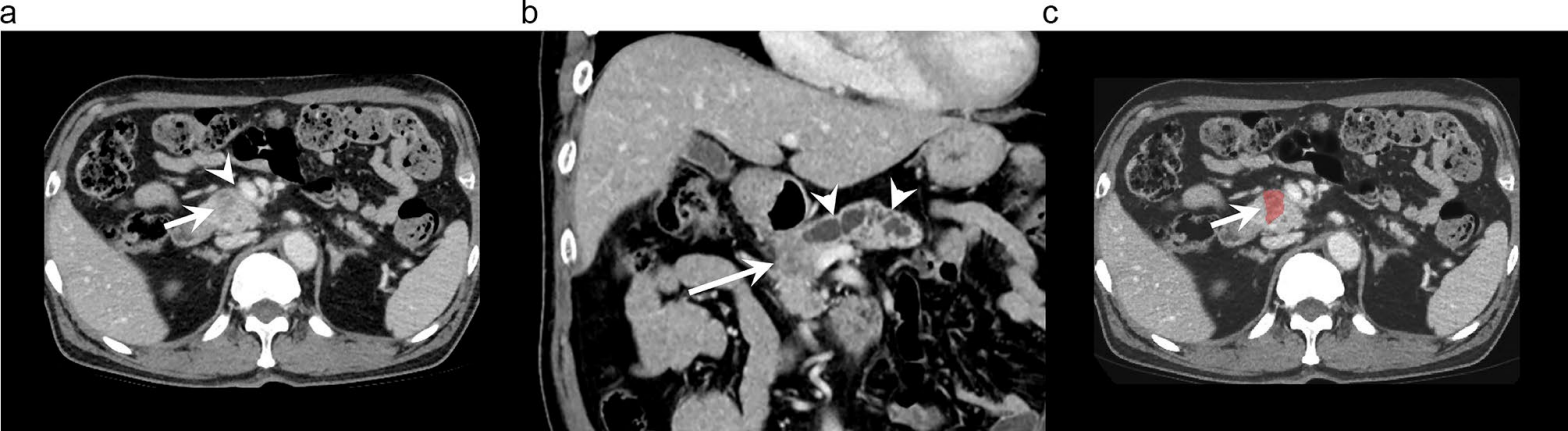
CT images of a 64-year-old male with pancreatic cancer. The patient’s preoperative CA19-9 level was 16.7 U/ml. (a) Axial portal venous phase CT image shows a 3.2 cm-sized pancreatic cancer (arrow) at the pancreas head. There was abutment of the tumor to the superior mesenteric vein (arrowhead), but no evidence of adjacent organ invasion. (b) Coronal portal venous phase shows the pancreatic cancer (arrow) with upstream main pancreatic duct dilatation (arrowheads). (c) Portal venous phase with segmentation overlay. An R0 resection was performed, with a pathological stage of T2N1. The CR model predicted non-early recurrence, while the CRR model predicted early recurrence. The patient experienced recurrence within 12 months, in the form of liver metastasis
